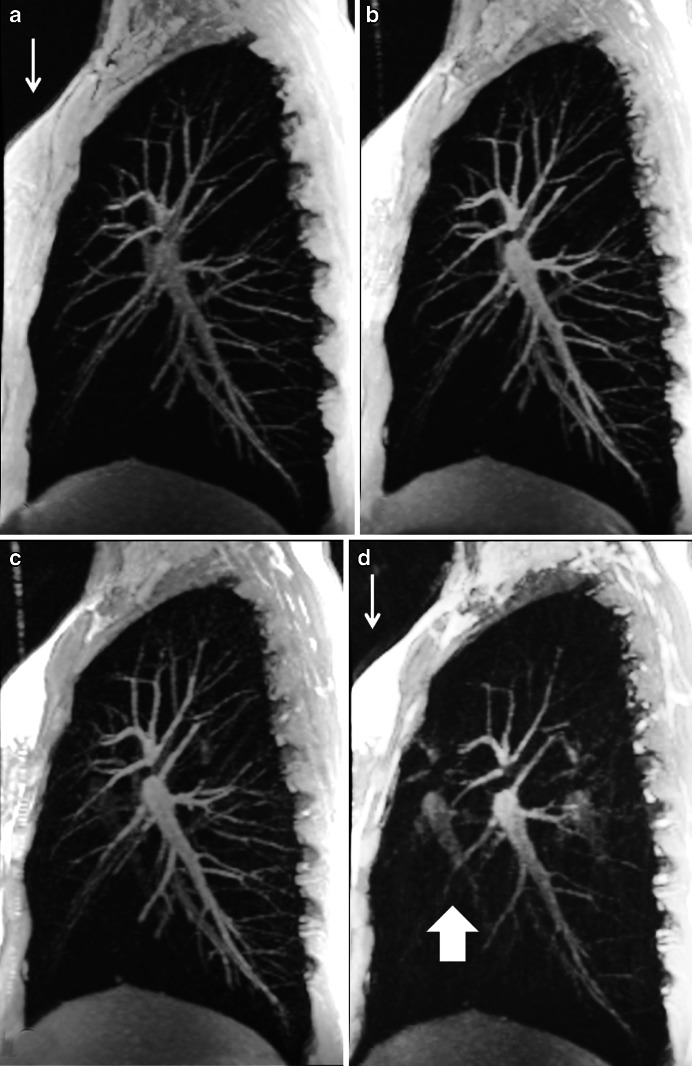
Time-of-flight angiography spoiled gradient echo in a 35-year-old male volunteer, acquired with increasing excitation flip angles: (a) 2°, (b) 6°, (c) 8° and (d) 12°. Note that by augmenting the flip angle the contrast shifts from proton density to T1-weighted, as observed on the fat signal intensity increase in the anterior chest wall (*arrows*). Higher flip angles provide better differentiation between arterial and venous signal at the expense of ghosting artifacts (*thick arrow*)
